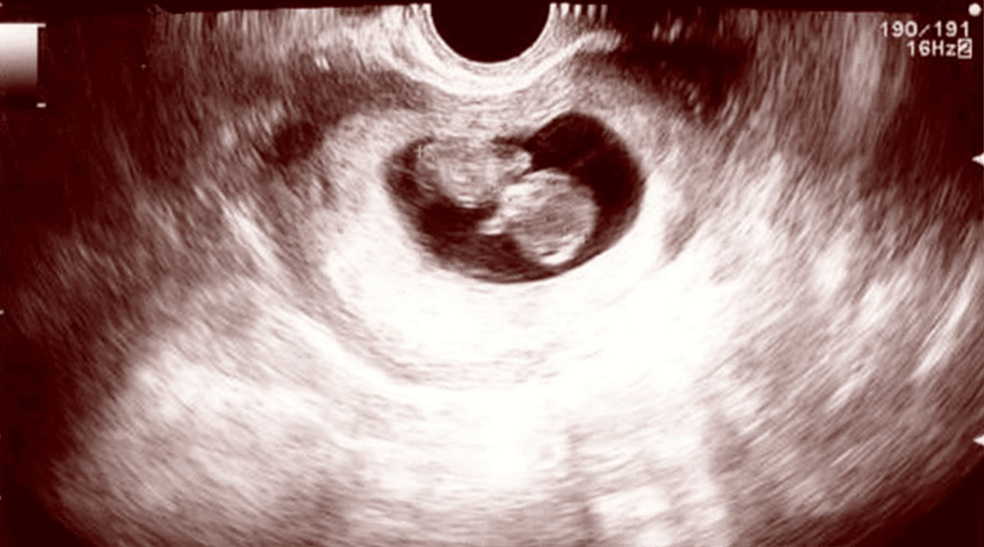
An ultrasound image of her pregnancy
